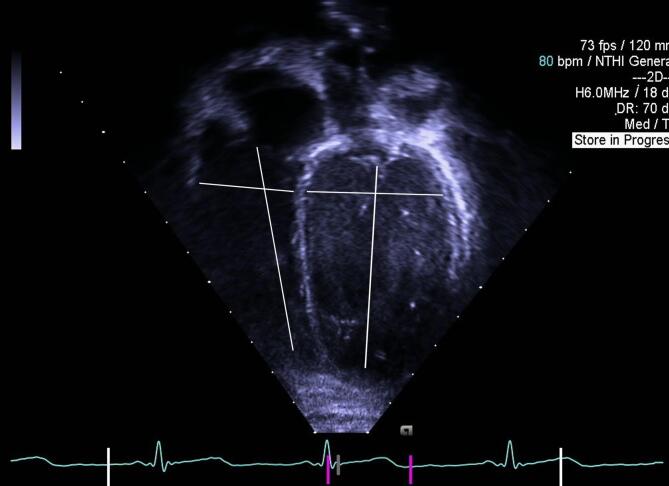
Measurements of longitudinal length and transverse width in end-diastole of left ventricle (LV) and right ventricle (RV) ffrom an apical 4-chamber view (Ap4C).
